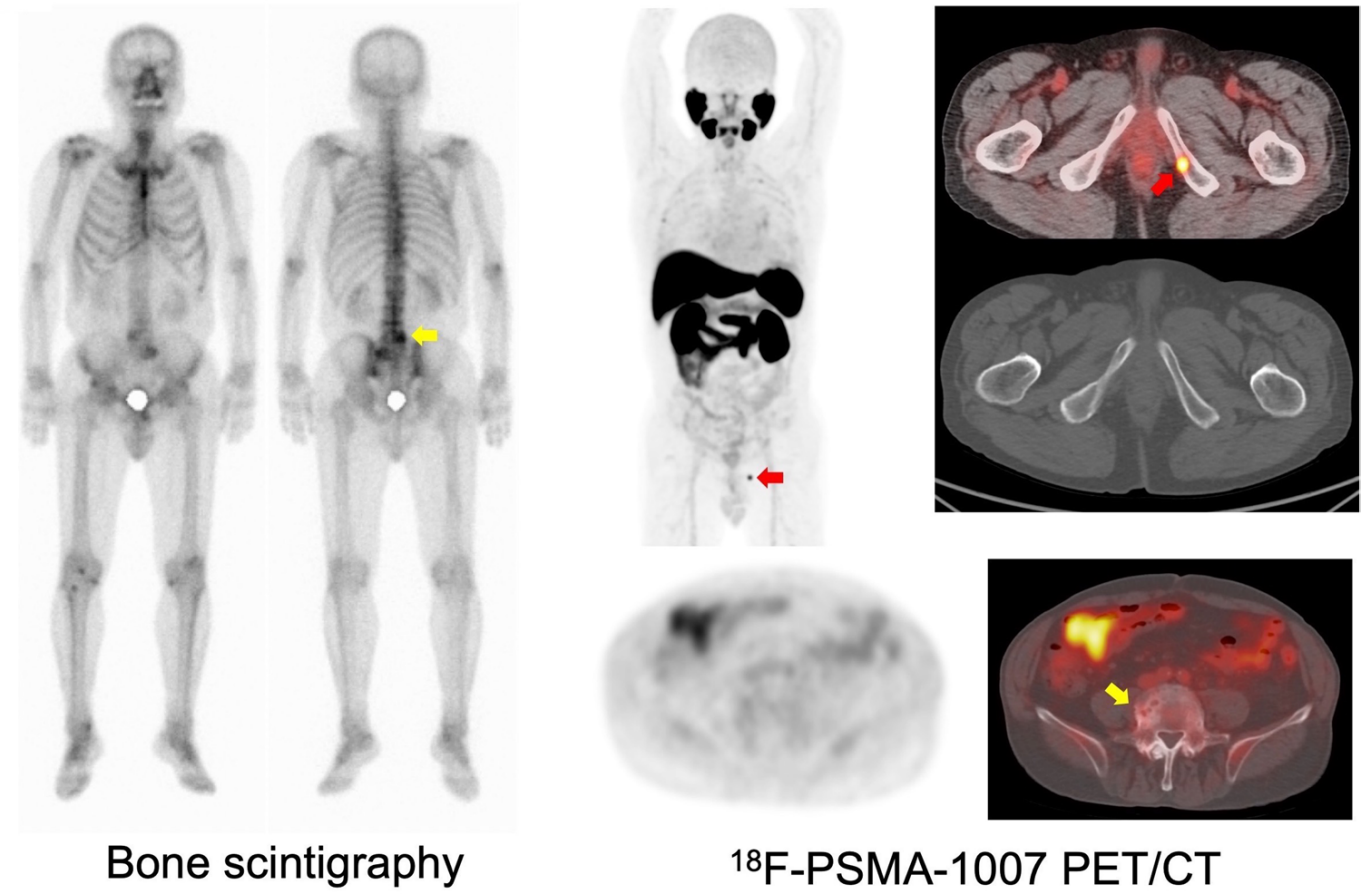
BCR patient after radical prostatectomy (PSA at PET: 1.7 ng/mL). Bone scintigraphy shows abnormal uptakes (yellow arrow) on the lower lumbar spine, which is considered to be a degenerative change. PSMA-PET shows focal uptake (red arrows) on the left ischium; whereas, it shows no significant uptake on the lower lumbar spine
